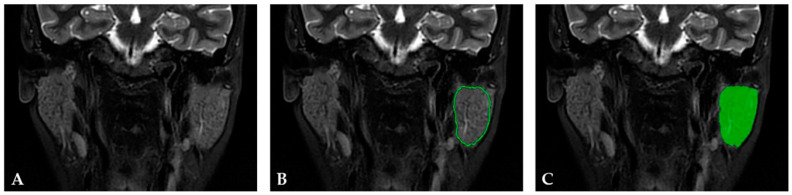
(A) MRI coronal STIR PROPELLER sequence of a 57-year-old female patient diagnosed with primary Sjögren’s syndrome. (B) The region of interest (ROI) covering almost the entire parotid gland was semiautomatically delineated by the software following geometry and gradient coordinates (green circle). (C) The final ROI after the manual correction was performed (green area).
